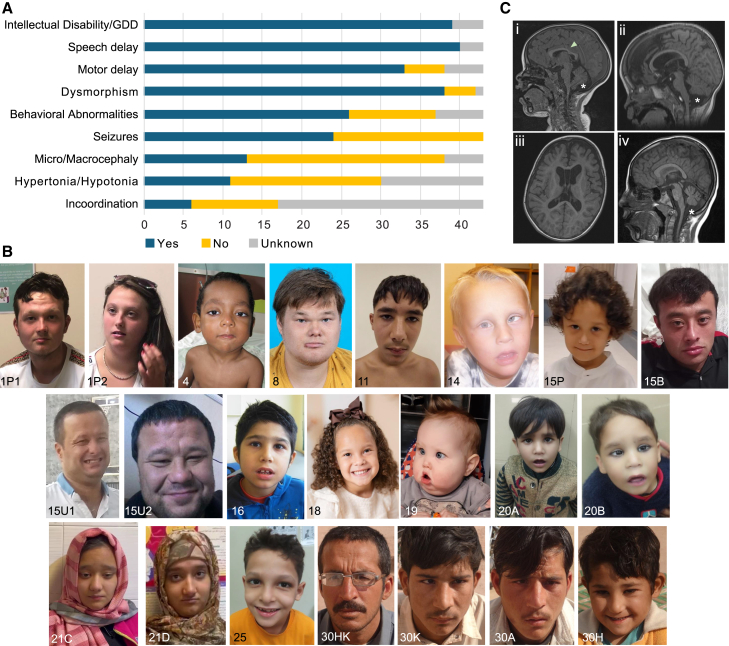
Genetic and phenotypic summary of the reported individuals with homozygous *TRMT1* variants (A) Clinical features of the affected individuals with bi-allelic *TRMT1* variants. GDD, global developmental delay. (B) Frontal facial photographs of *TRMT1* probands showing the most prominent and frequent dysmorphic features of *TRMT1*-related neurodevelopmental delay. (C) Representative neuroimaging features identified in individuals with intellectual disability. (i) Midsagittal T1-weighted MRI of the brain in a four-year-old boy (F-5) exhibits global (cerebral and cerebellar) atrophy, posterior thinning of the corpus callosum (arrow), and a mega cisterna magna (asterisk). (ii and iii) Midsagittal (ii) and axial (iii) T1-weighted MRI of the brain in a 4-year-old boy (F-8) shows further characteristic features of intellectual disability associated with *TRMT1*, namely, frontotemporal-predominant cerebral and midbrain atrophy with corresponding ventriculomegaly and uniform thinning of the corpus callosum (not all shown). Note is also made of the right posterior positional plagiocephaly. (iv) Midsagittal T1-weighted brain MRI of 7-year-old boy (F-23) exhibits cerebellar atrophy, a mega cisterna magna (asterisk), and downsloping of the corpus callosum.
